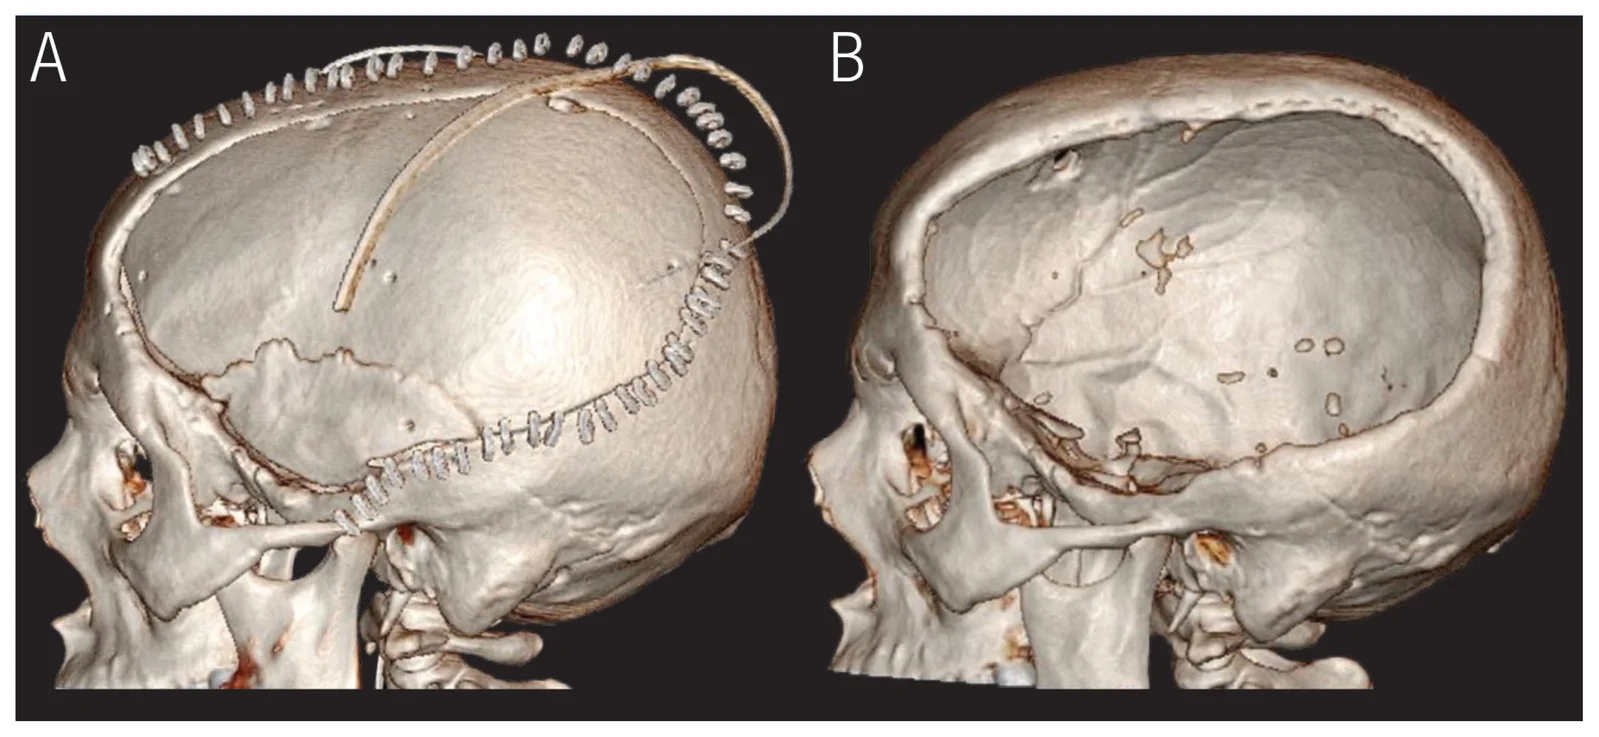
A computed tomography scan with 3D reconstruction of the right side of the skull (A) third-day post cranioplasty with bone graft replacement (bone from the previous craniectomy) and (B) 9 months post-operative showing severe resorption of the bone graft (>98).
